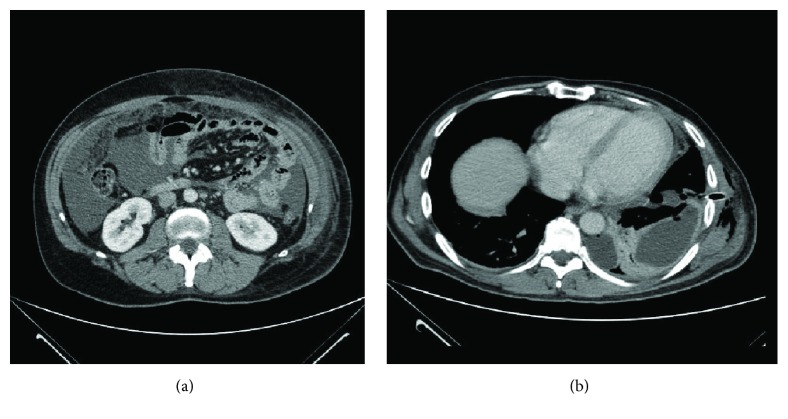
(a) At the time of diagnosis diffuse peritoneal fluid in the abdomen and peritoneal implants are presented through the CT scan. (b) Pleural effusion on the left (progressive disease) presented after the third cycle of Capecitabine/Oxaliplatin chemotherapy.
